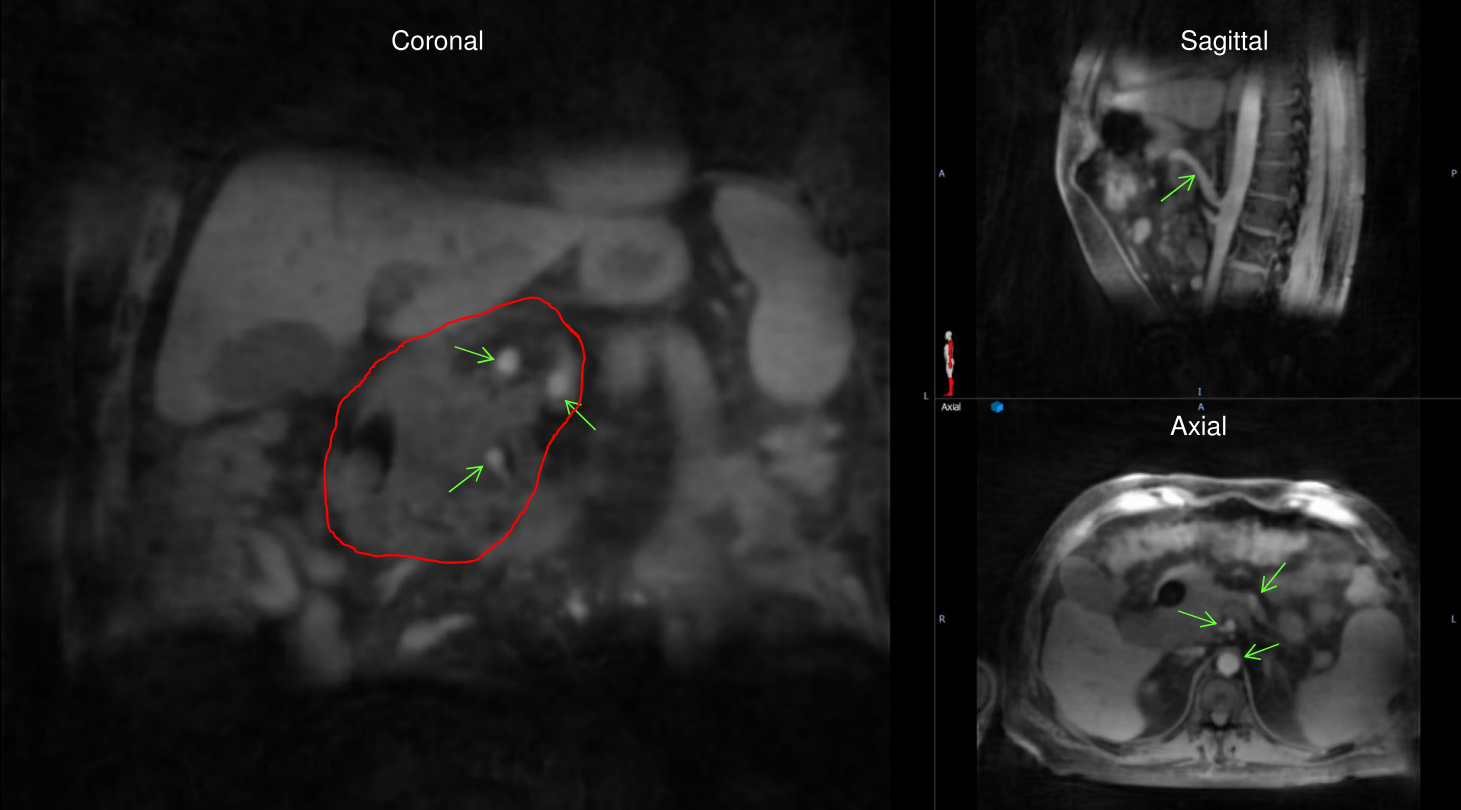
Example 4D-MRI images in coronal, sagittal and axial planes showing the tumor (red circle) and infiltrated blood vessels (green arrows)
